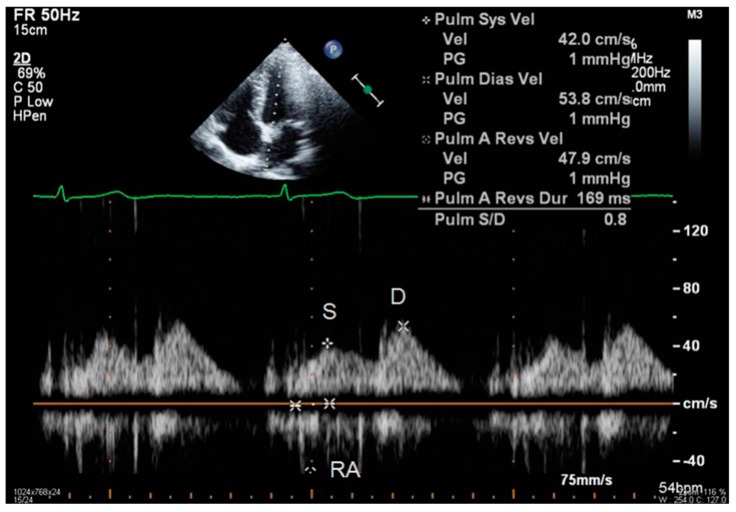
Echocardiographic measurement of S and D wave peak velocity by pulsed Doppler on right superior pulmonary vein flow. D: peak velocity of diastolic wave: RA: peak velocity of reverse atrial wave; peak S: peak velocity of systolic wave.
